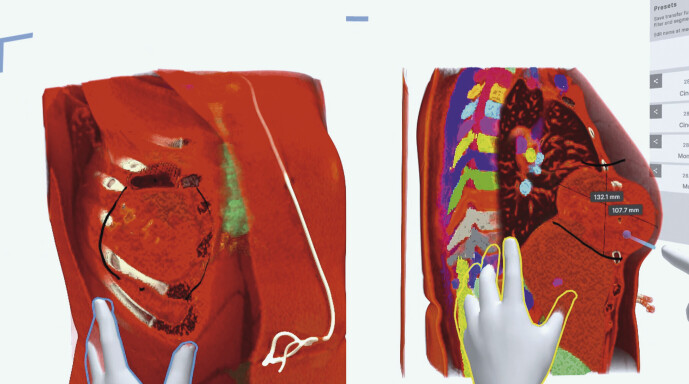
VR-reconstruction of a CT-scan showing a chest wall tumor with interactive measurement tools used in the interdisciplinary retrospective discussion.
